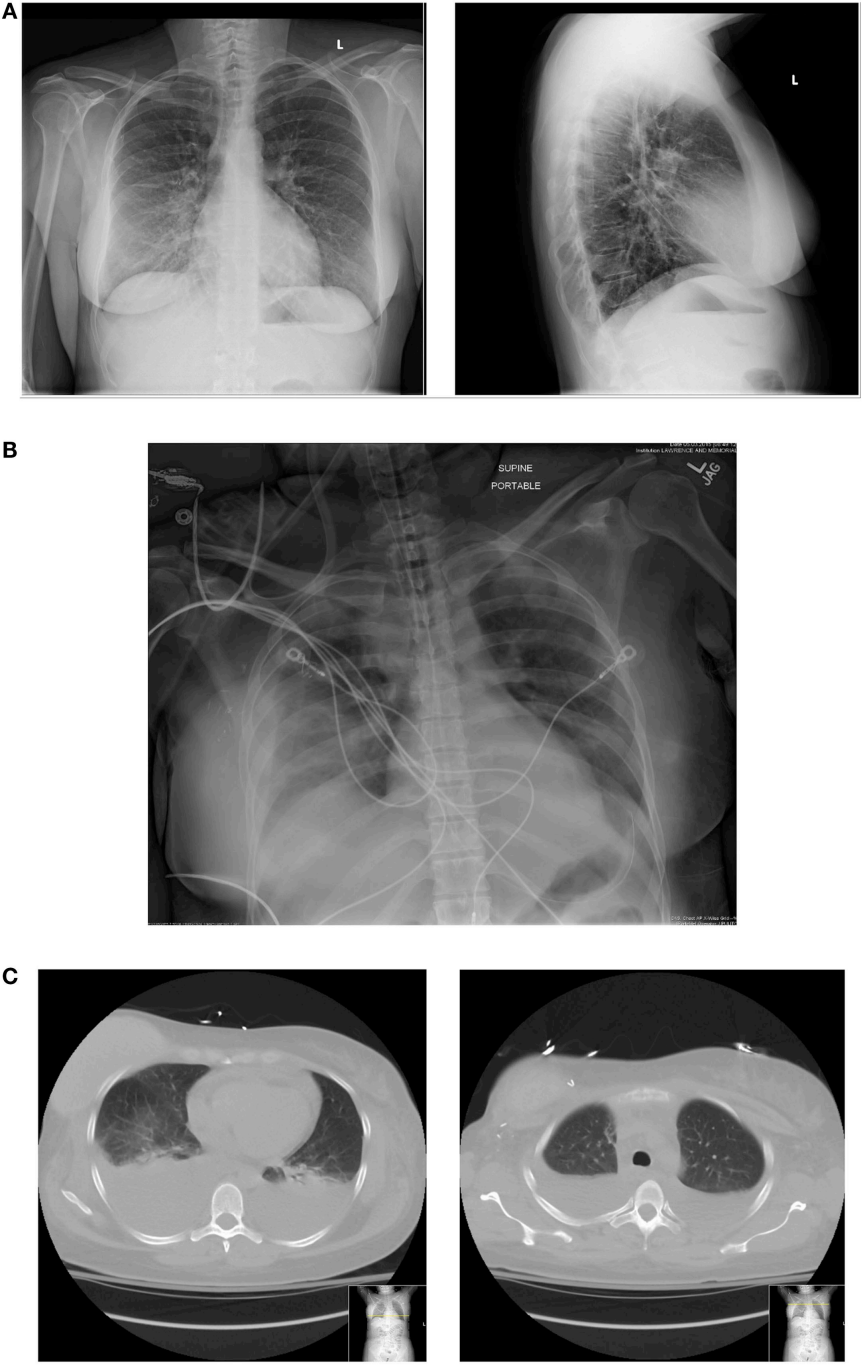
(A) Initial chest radiograph. (B) Chest radiograph 48 h at ICU admission. (C) Corresponding CT chest with representative cuts at bases (just above diaphragm) and upper-chest showing large pleural effusions and some adjacent compressive atelectasis but little/no parenchymal infiltrate.
